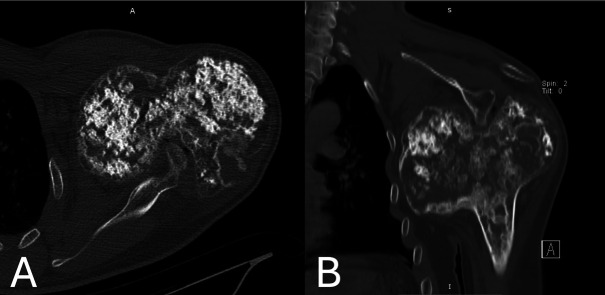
A & B. Computed tomography scan images showing the presence of extensive cartilaginous calcifications, cortical remodeling, and an osteolytic area with cortical disruption at the posterior aspect of the epiphyseal overgrowth. The medial epiphyseal overgrowth extended to the maxillary recess, whereas the glenoid process was seen to indent to the posterior aspect of the epiphysis.
